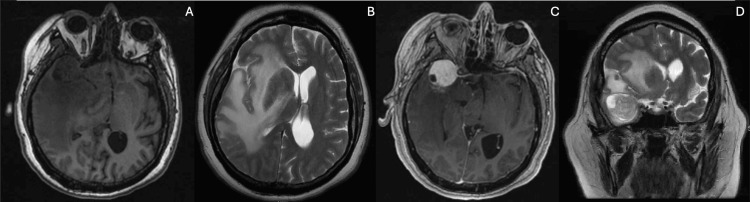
Right sphenoid wing meningioma observed on contrasted MRI. There is a rounded lesion at the level of the right sphenoid wing. It appears isointense and hypointense on T1 (A), and noticeable vasogenic perilesional edema is visible on T2 (B), causing a midline shift of more than 1 cm. The lesion shows intense and homogeneous enhancement with contrast (C). The midline shift caused by the vasogenic edema due to the right sphenoid wing meningioma is more clearly observed (D).
